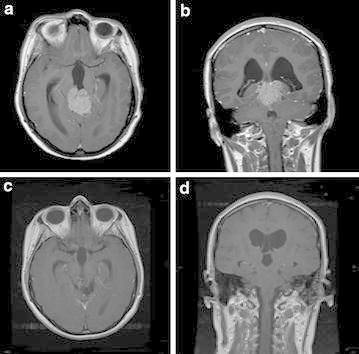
Patient with good PR (partial response). Axial and coronal T1-weighted MRI scans with intravenous Gadolinium, before (a and b) and after (c and d) treatment
